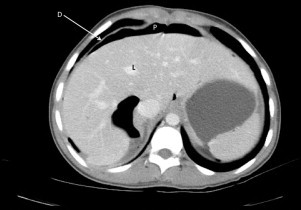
Axial CT slice of upper abdomen demonstrating a pneumopertioneum (P) that is contained by the diaphragm (D) and liver (L).
